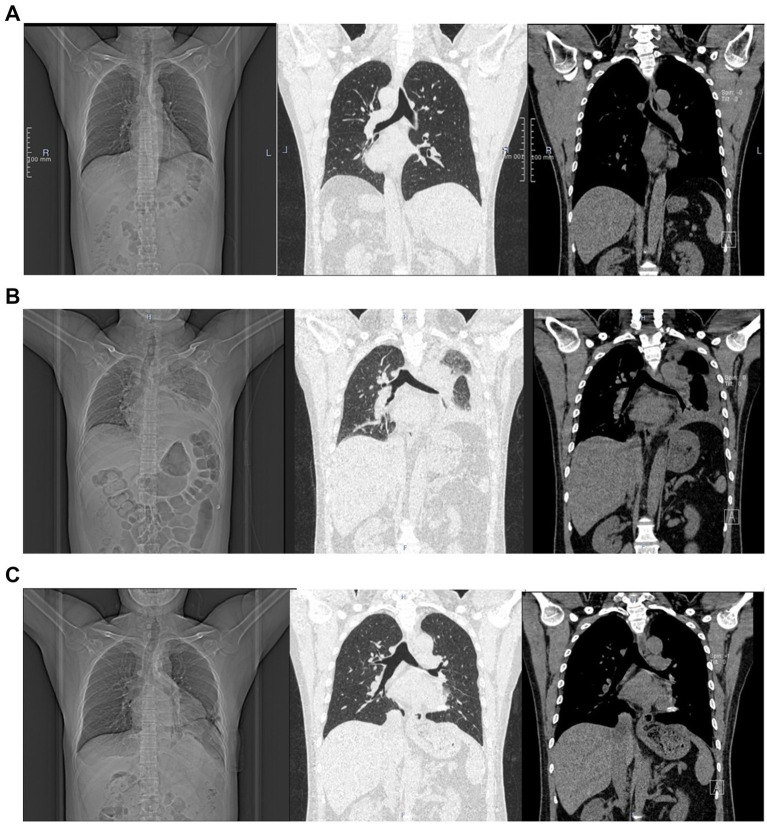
Radiological findings. (A) Preoperative chest CT obtained prior to thymectomy demonstrates normal bilateral diaphragmatic position, fully expanded lungs, and absence of gastric distension. (B) Post-thymectomy imaging at emergency presentation (postoperative day 10) demonstrates marked elevation of the left hemidiaphragm, complete left lower lobe atelectasis, and massive gastric distension compressing the left hemithorax; CT pulmonary angiography confirmed the absence of pulmonary embolism. (C) Chest CT obtained 48 h after single-incision VATS diaphragmatic plication demonstrating complete left lung re-expansion, restoration of diaphragmatic position at the level of the posterior eighth rib, and resolution of gastric distension.
